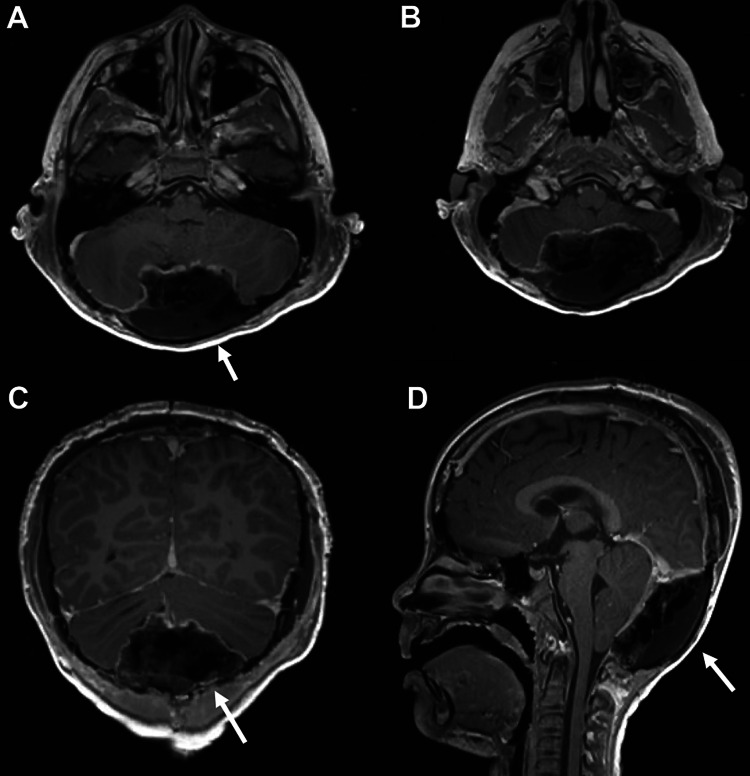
Postoperative magnetic resonance imaging demonstrating gross total resection of the tumor. (A, B) Axial plane. (C) Coronal plane. (D) Sagittal plane.
